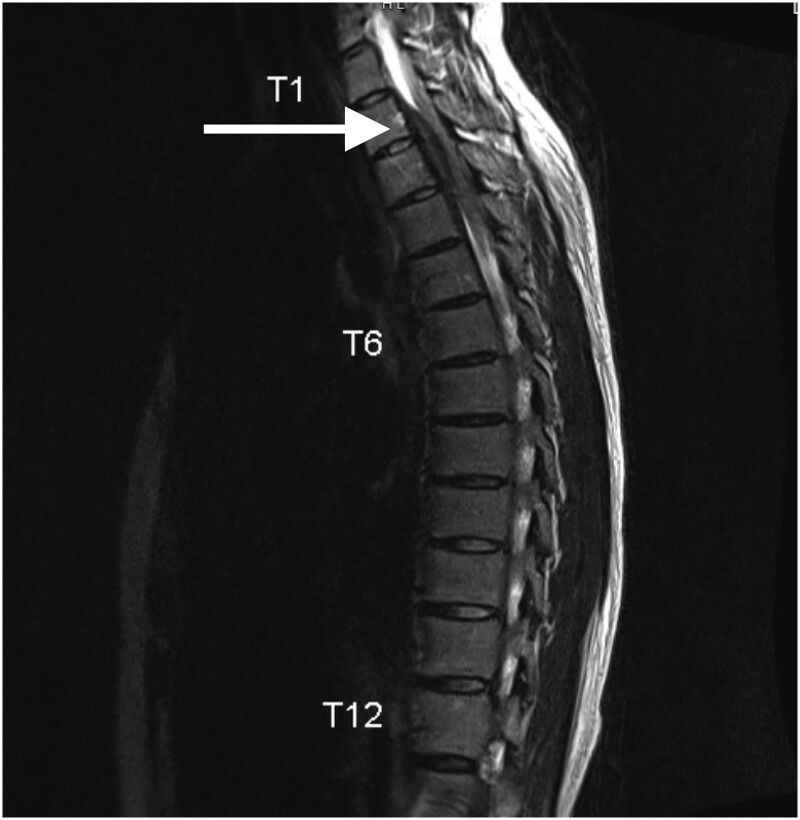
Initial magnetic resonance imaging of the thoracic spine showing prominent T2 to T4 stenosis from unknown soft tissue mass, sagittal view.
